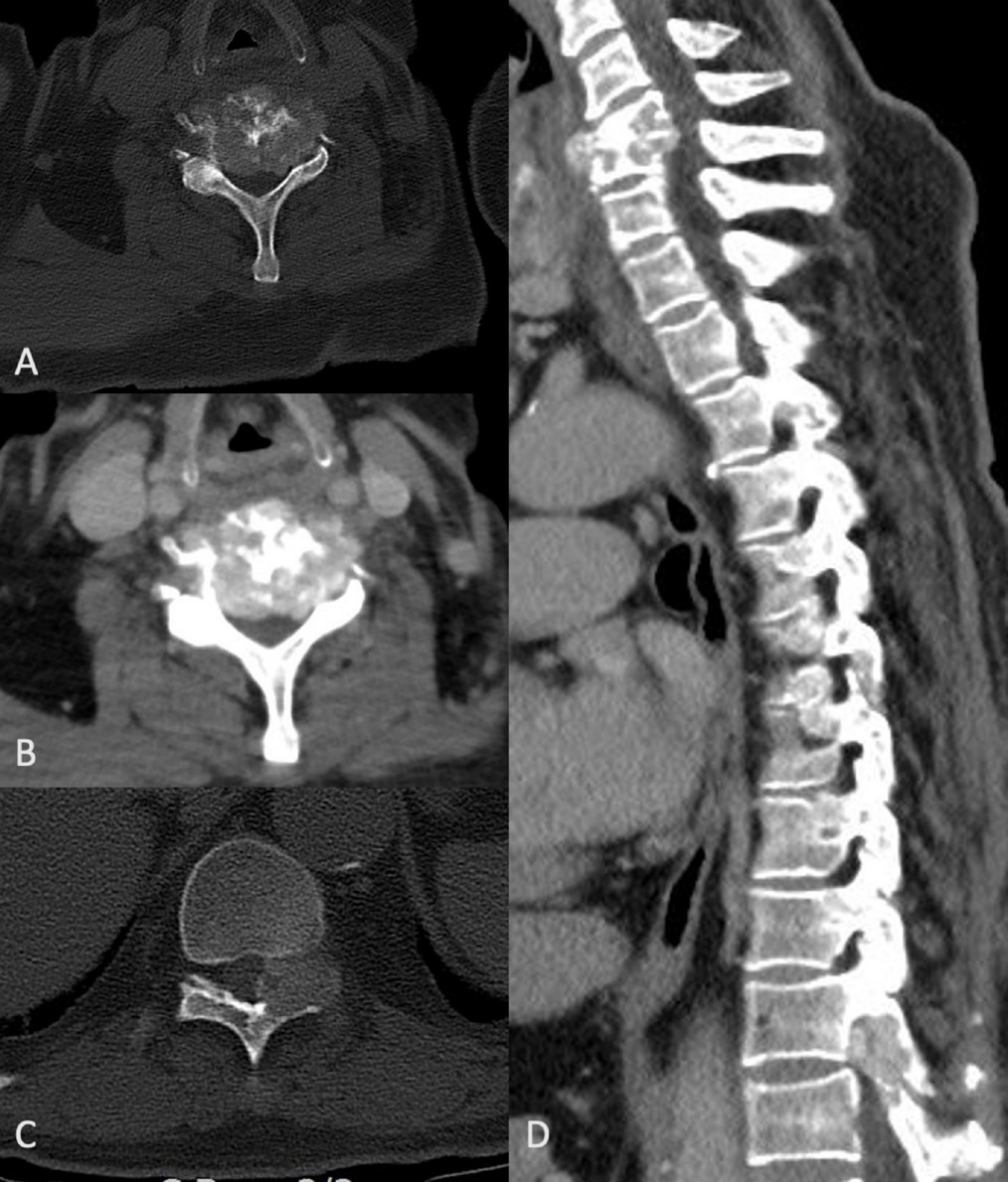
A, axial bone window CT image showing bulging soft tissue mass causing narrowing of spinal canal and obliteration of bilateral neural foramina at the C5 level; B, post contrast CT image showing avid enhancement of the soft tissue; C, another soft tissue lesion at the left pedicle of T10 vertebra causing narrowing of the spinal canal; D, sagittal reformatted images showing multi-level involvement
